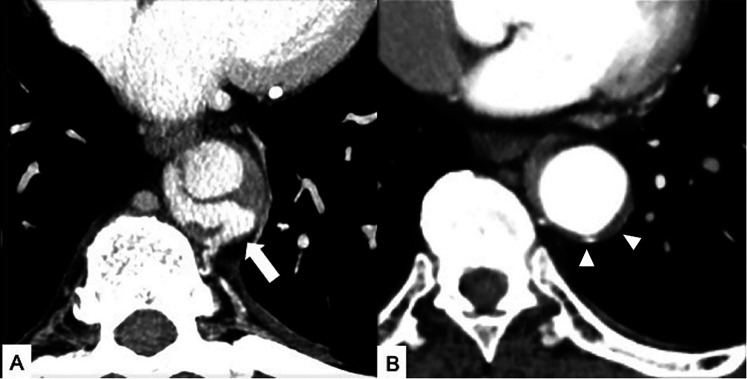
Intramural blood pool (IBP) in a 61-year-old patient with systemic hypertension and Type B thoracoabdominal IMH and acute back pain. (A) Axial CT maximum intensity projection (MIP) reconstruction image shows a T9 level intercostal artery pseudoaneurysm (arrow) in the contest of the Type B IMH with absent communication with the true aortic lumen. (B) Enhanced CT axial image obtained 3 months later shows a partial IBP and IMH regression (arrowheads).
